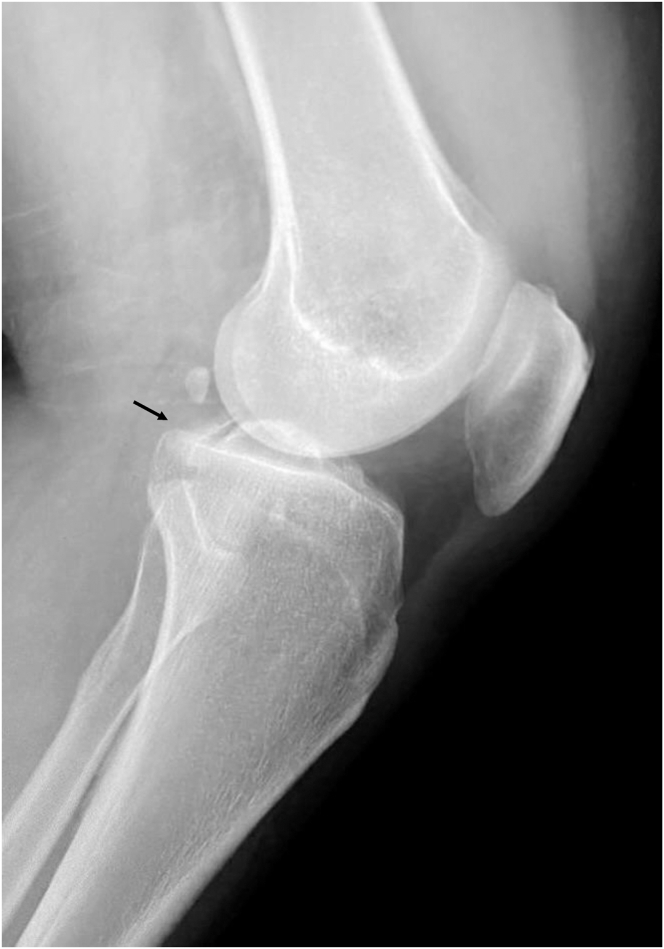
Lateral view radiograph of the right knee, showing a displaced posterior cruciate ligament tibial avulsion fracture injury. In the presence of minimally displaced fractures, fluoroscopic stress views can be obtained to evaluate for posterior cruciate ligament laxity.
